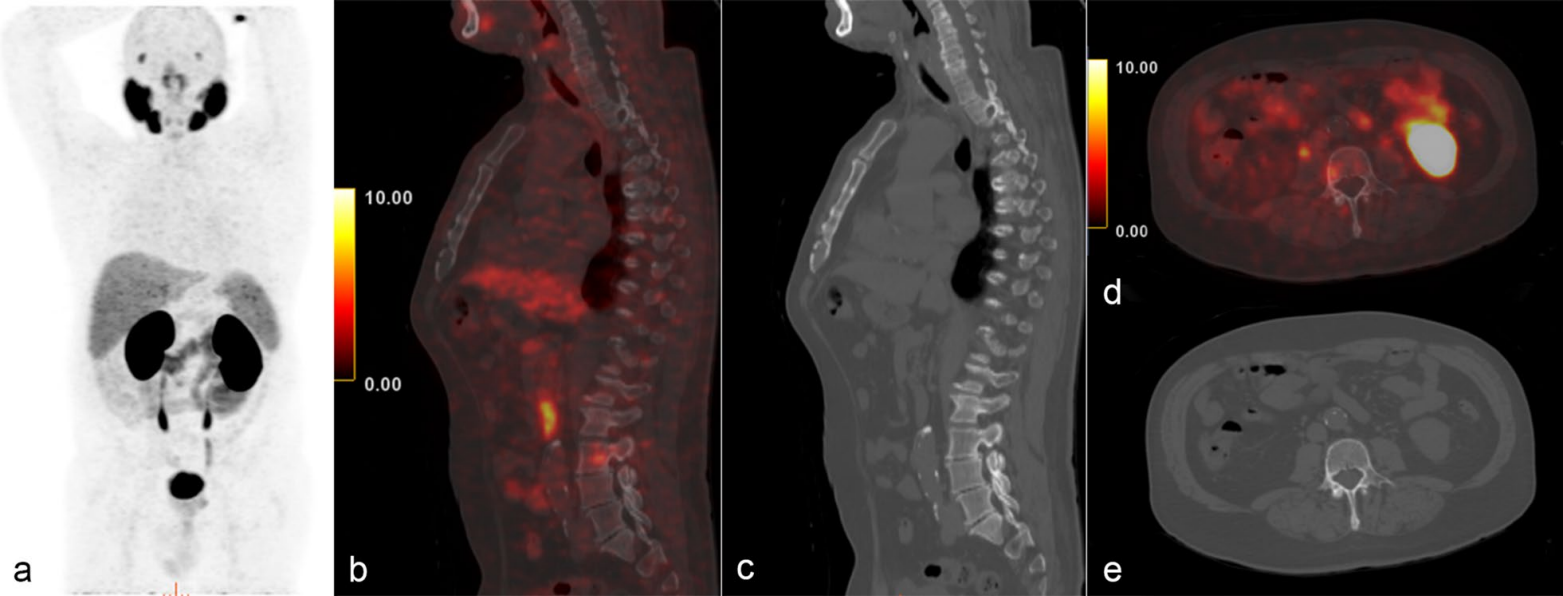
A 74-year-old patient (patient number 14) with unfavorable intermediate risk PC. Aside from the primary prostatic lesion, focal mild uptake in L3 vertebral body was identified on staging 68 Ga-PSMA-11 PET/CT, with no pathological findings on CT, categorized as a PSMA-RADS 3B lesion. This lesion was deemed as false-positive by Post-RP PSA measurements and clinical follow-up. Staging PET/CT mip (a); sagittal fusion (b) and CT (c); axial fusion (d) and CT (e)
